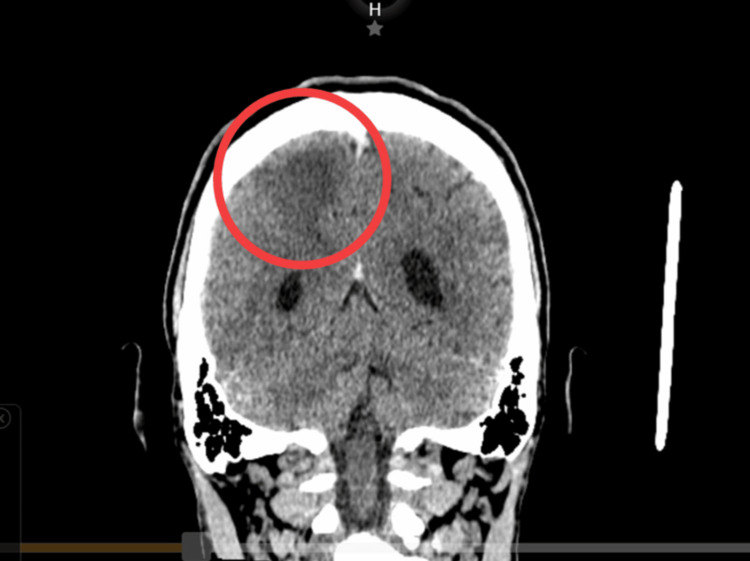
CT scan of the patient's skull (coronal view) Simple coronal skull CT scan with report of ischemic cerebrovascular event in the right parietal lobe, including the semioval center. The red circle indicates the presence of a hypodense lesion consistent with an ischemic cerebrovascular event in the right parietal lobe, including the semioval center.
